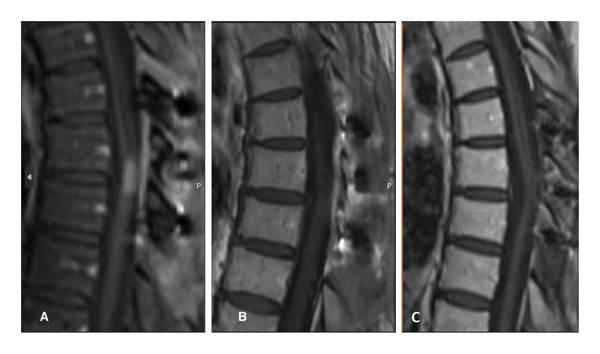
Sagittal T1 postcontrast MRI 1 month after radiation therapy (A) reveals persistent intramedullary enhancement that demonstrates a significant treatment response 2 months (B) and 18 months (C) after initiating alectinib therapy.
